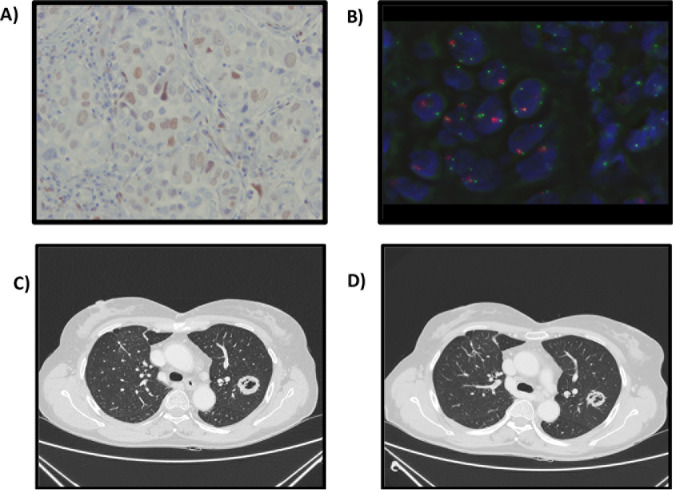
Biological and radiological features: A) Estrogen Receptor, B) FGFR2 Amplification; C) Target lesion at basal CT scan, D) Target lesion after 12 months of treatment
